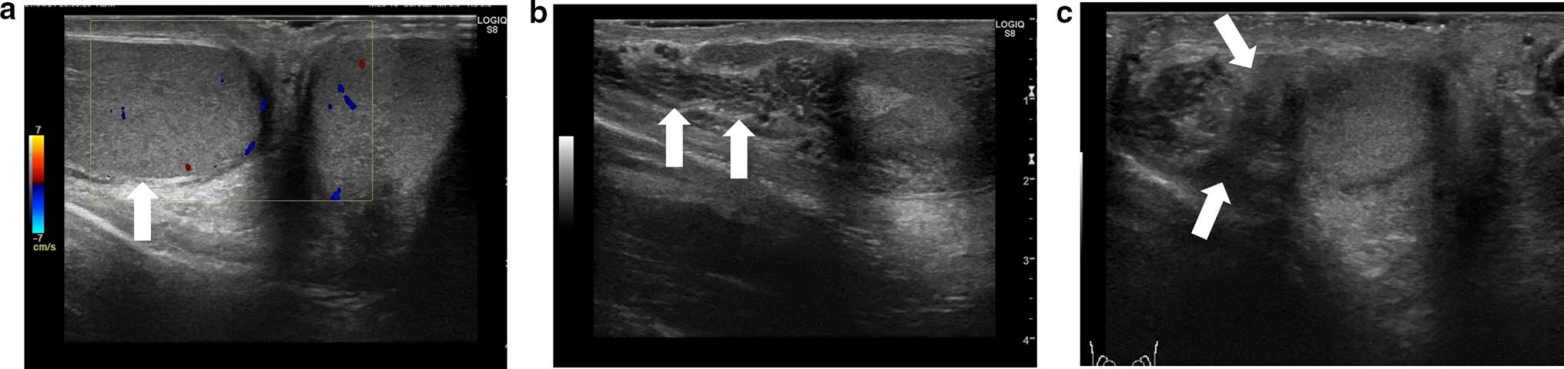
Ultrasonographic images of a 13-year-old boy with right intermitted testicular torsion. a The right testis has an abnormal axis and decreased blood flow. b The spermatic cord is redundant, but the whirlpool sing is absent. c A pseudomass is present adjacent to the testis. Intermitted testicular torsion was diagnosed based on these findings
